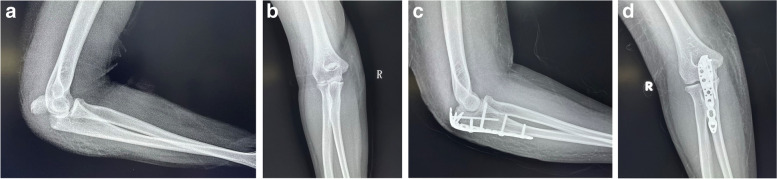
A 47-year-old male underwent surgery with 3.5 mm LCP. a b. Anteroposterior and lateral X-ray film of the elbow joint before operation. c d. Postoperative anteroposterior and lateral X-ray film of the elbow joint
